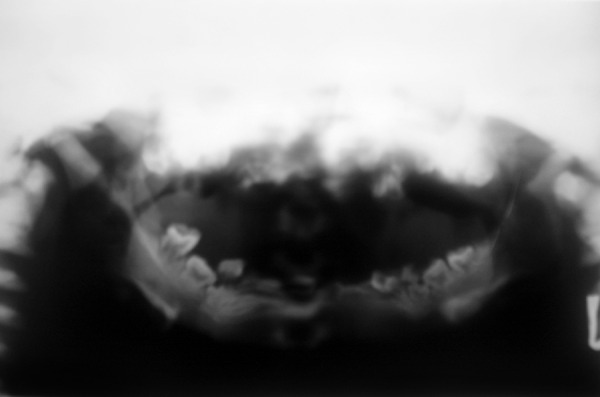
Panoramic radiographs showing indistinct head of condyle and coronoid process, with an increased gonial angle, diffuse radiopacity of the jaw
